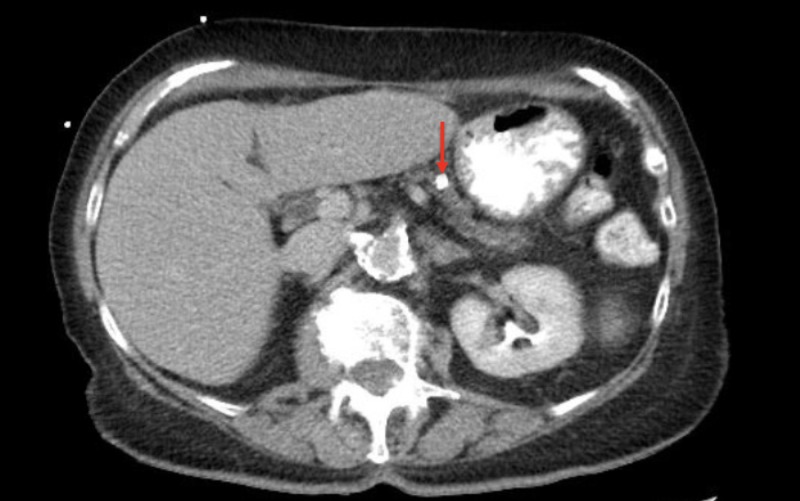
Computed tomography of the abdomen in transverse view showing a 10 mm pancreatic calculus (red arrow)
